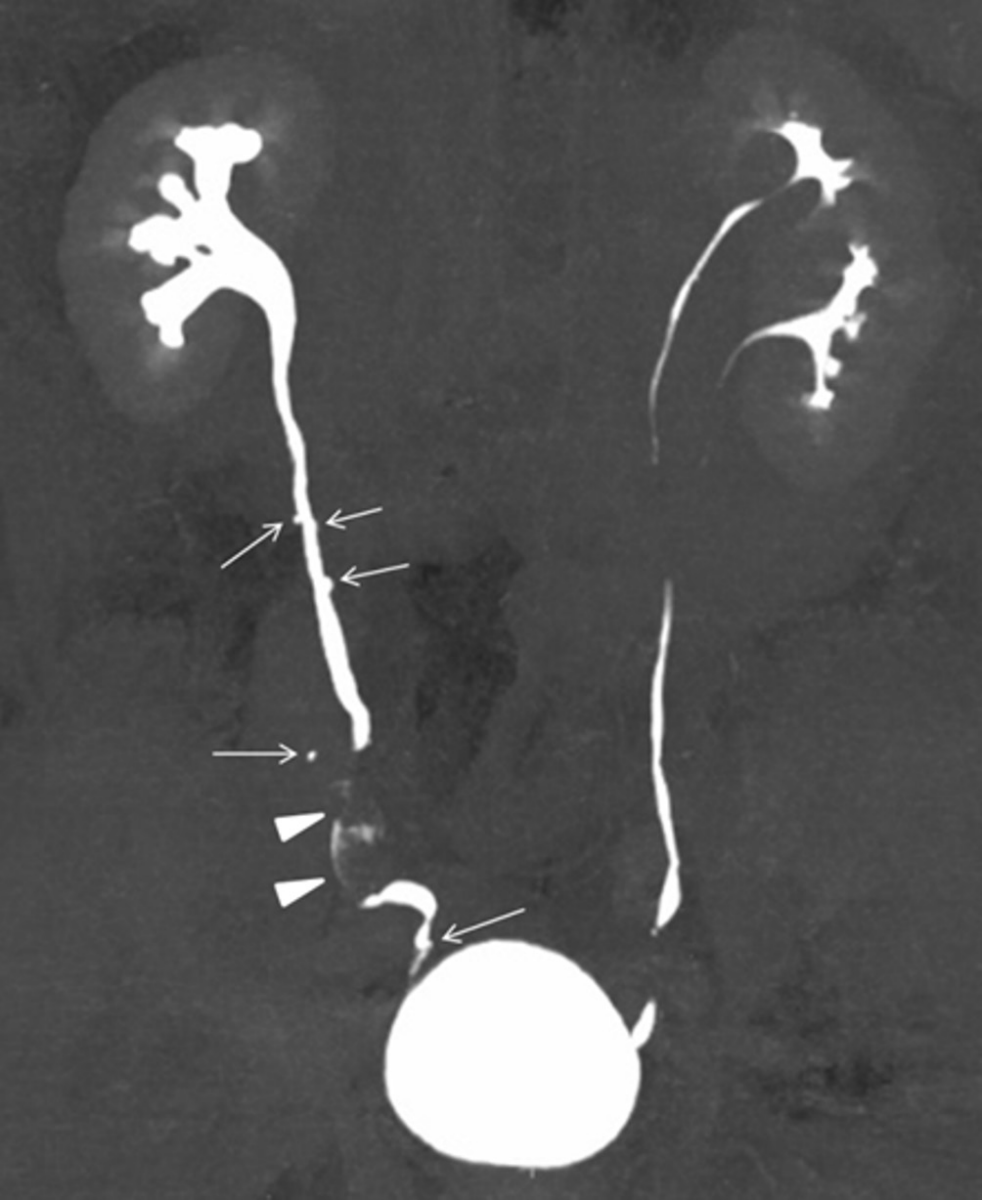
CT urography (excretory phase/maximum intensity projection image) While mild right hydronephrosis was present, the excretion of the contrast medium was satisfactory. Multiple outpouchings of contrast medium measuring a few millimetres (arrows) are visible in the right ureter, suggesting ureteral pseudodiverticulosis. The arrowhead indicates a filling defect due to the right middle ureteral cancer. Incomplete double renal pelvis and ureter on the left side are also visible.
